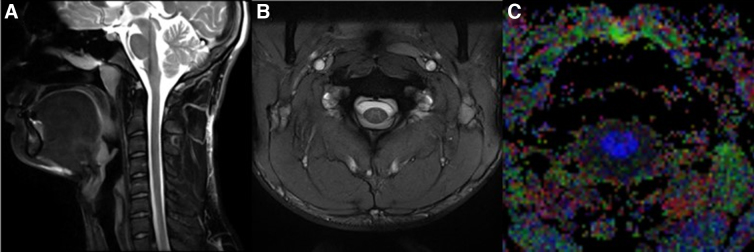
Acquired scans. (A) 2D sagittal T2-weighted fat saturated turbo spin echo, (B) 3D axial T2*-weighted multi-echo gradient echo, and (C) 2D axial diffusion tensor imaging. 2D, two-dimensional; 3D, three-dimensional.
